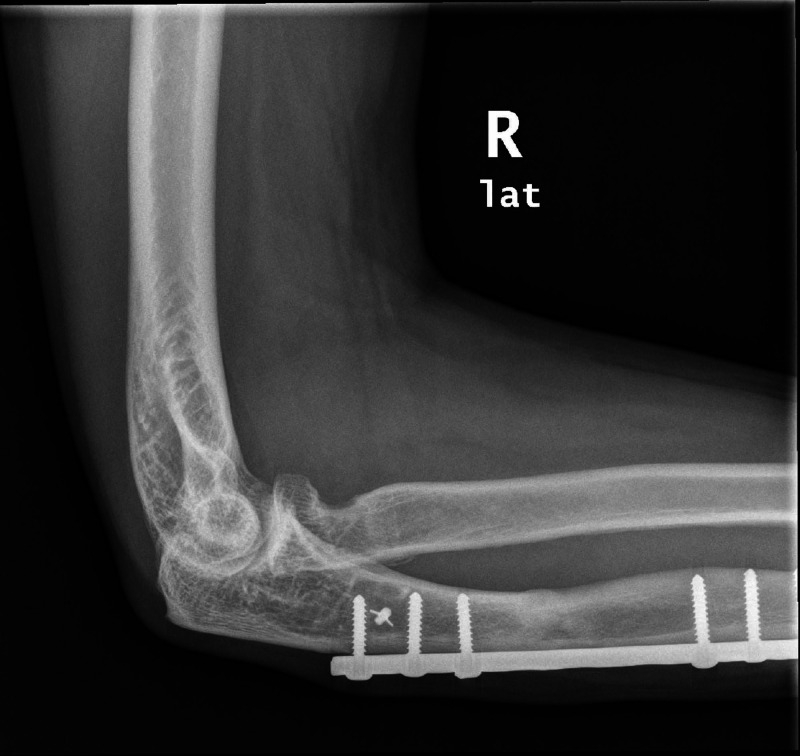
Lateral elbow radiograph two years post-annular ligament reconstruction with osteolysis of the radial head.
